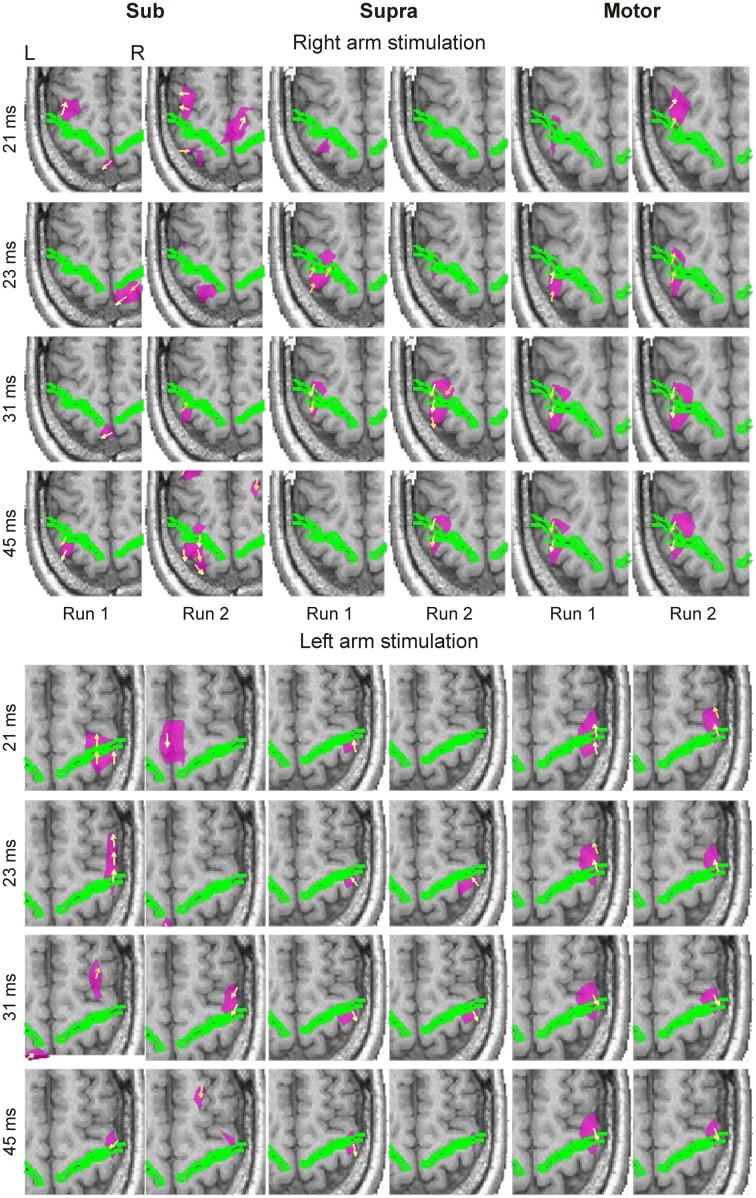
Averaged spatio-temporal CDV-maps of the blind subject are shown for both runs of Sub (columns 1 and 2), Supra (columns 3 and 4) and Motor (columns 5 and 6) intensities at four latencies (indicated at the left side of each row) in response to right (upper block) and left (lower block) arm stimulations. The axial slice of MRI best covering the activated part of central sulcus, indicated by a green outline, is shown. Yellow arrows show the direction of CDV. Pink contours encompass regions where the normalized magnitude of CDV is above 0.9. For display purposes the CDV magnitudes were normalized independently for each image. This made all the activations clearly visible. L, left; R, right.
